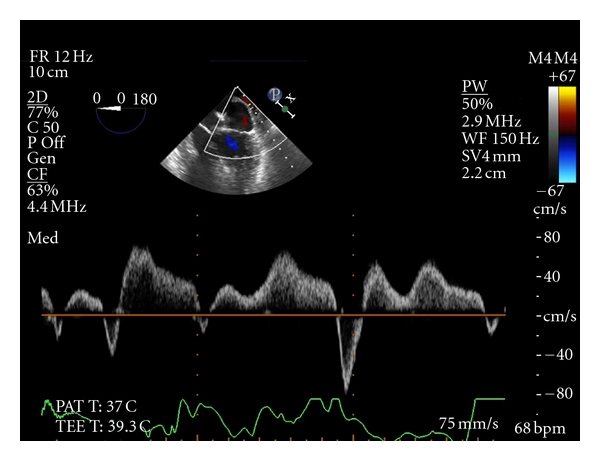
Pulse wave Doppler interrogation of the left superior pulmonary vein. Ar wave of 80 cm/sec is noted.
